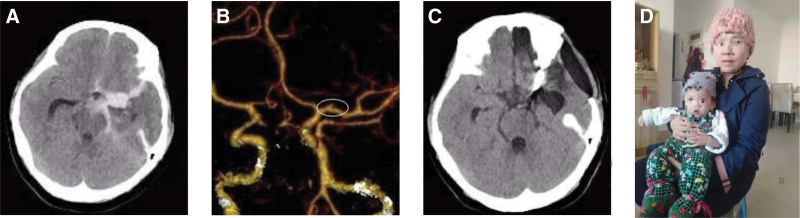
The second trimester of pregnancy complicated with a ruptured left middle cerebral aneurysm. (A) Preoperative brain CT showed patchy and banded high-density shadow around the middle cerebral artery, lateral fissure cistern, tentorium of cerebellum, and adjacent sulcus on the right, and high-density filling in the left lateral ventricle, third ventricle, and fourth ventricle. (B) Craniocerebral CTA showed a bag-like high-density shadow in the proximal segment of the left middle cerebral artery; bilateral anterior, middle, and posterior cerebral arteries were roughly symmetrical; and no obvious abnormality was found in the vertebrobasilar artery. (C) Brain CT reexamination after operation. (D) Postoperative follow-up showed that the mother and child were healthy. CT = computed tomography, CTA = computed tomography angiography.
